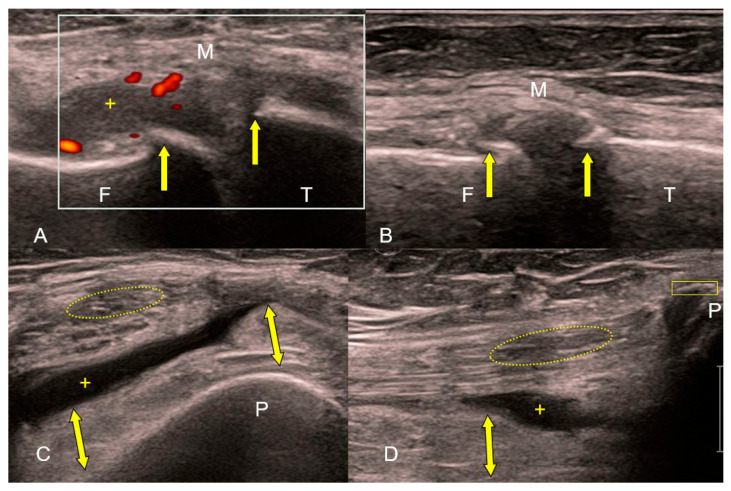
(A–D) Ultrasound of the knee joint in exacerbation of osteoarthritis (high-frequency linear probe: B-mode and Power Doppler). Irregular echoes from the femoral (F) and tibial (T) articular surfaces corresponding to marginal osteophytes (A,B). Hyperemia (red color in white rectangle) and edema of the thickened, hypertrophic synovial membrane (yellow bidirectional arrows) with effusion (+) in the suprapatellar bursa are signs of reactive inflammation. Loss of tension and fine linear echostructure of the medial collateral ligament (M). Absence of linear echostructure and decreased echogenicity (yellow ellipse) in the distal part of the quadriceps muscle tendon. Enthesophyte (yellow rectangle) at the base of the patella (P) (C,D).
